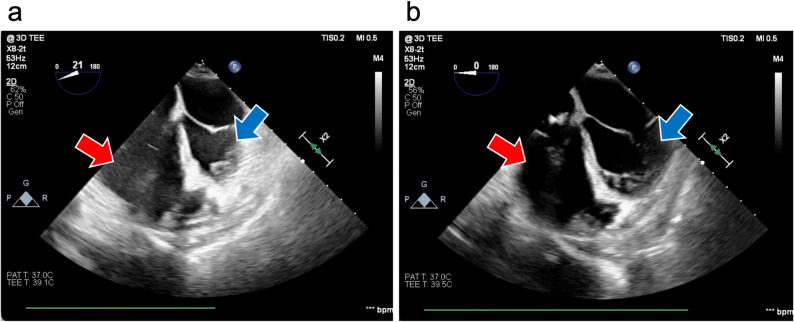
Mid-esophageal four-chamber views on transesophageal echocardiography. a: During anaphylactic shock refractory to standard therapy, the interventricular septum was displaced leftward, with right ventricular dilatation (red arrow) and reduced left ventricular size (blue arrow), findings consistent with severe right heart failure. b: Echocardiographic findings 30 min after initiating dobutamine, olprinone, and inhaled nitric oxide therapy; the right ventricular dilatation is reduced (red arrow), and left ventricular filling has been achieved (blue arrow), confirming resolution of acute right heart failure
